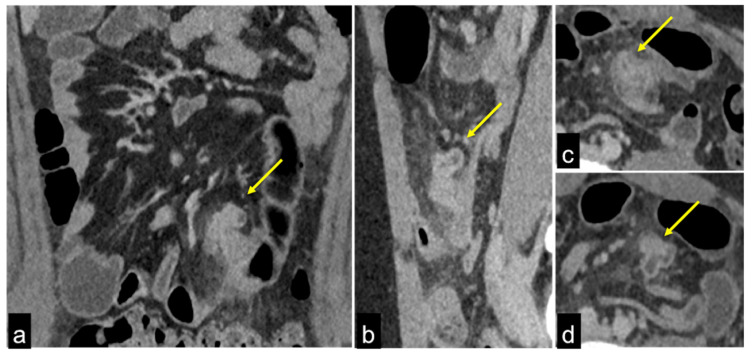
Fifteen-year-old patient complaining of abdominal pain and anaemia. Enhanced CTA scan in portal venous phase in coronal (a), sagittal (b), axial (c,d) views. In the mesogastric region, there is a diverticular ileal structure (a–d, arrows) with increased wall enhancement at CTA venous phase, associated with adjacent fat stranding. Findings are consistent with Meckel diverticulitis.
